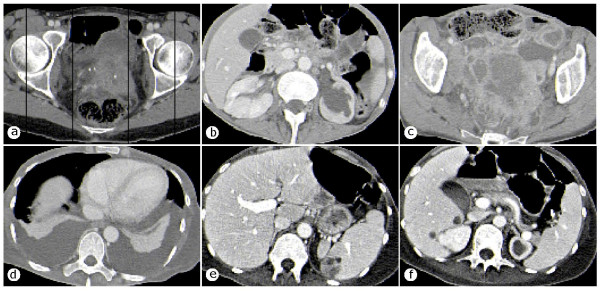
CT scans. (a) local tumor progression in her pelvis and vesical invasion and hemorrhage; (b) left ureter obstruction; (c) ileus through descending colon and rectosigmoid obstruction; (d) malignant pleural effusion; (e) spleen metastasis; (f) liver metastasis.
